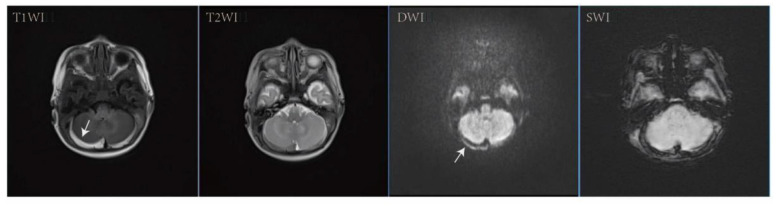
MRI images of subarachnoid hemorrhage. T1WI showed increased signal intensity in sulcus for occipital sinus. T2WI and DWI showed decreased or loss of signal intensity in the same localization.
